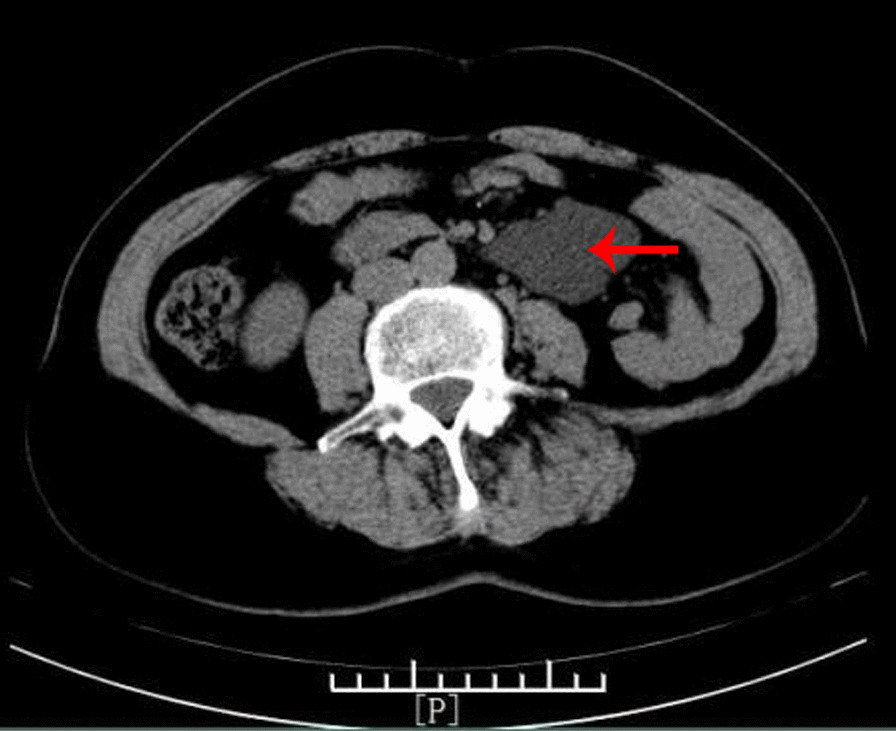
Plain abdominal CT scan showing an abdominally irregular low-density mass of 5.9 cm × 4.1 cm on October 25, 2020 (Case 2)
